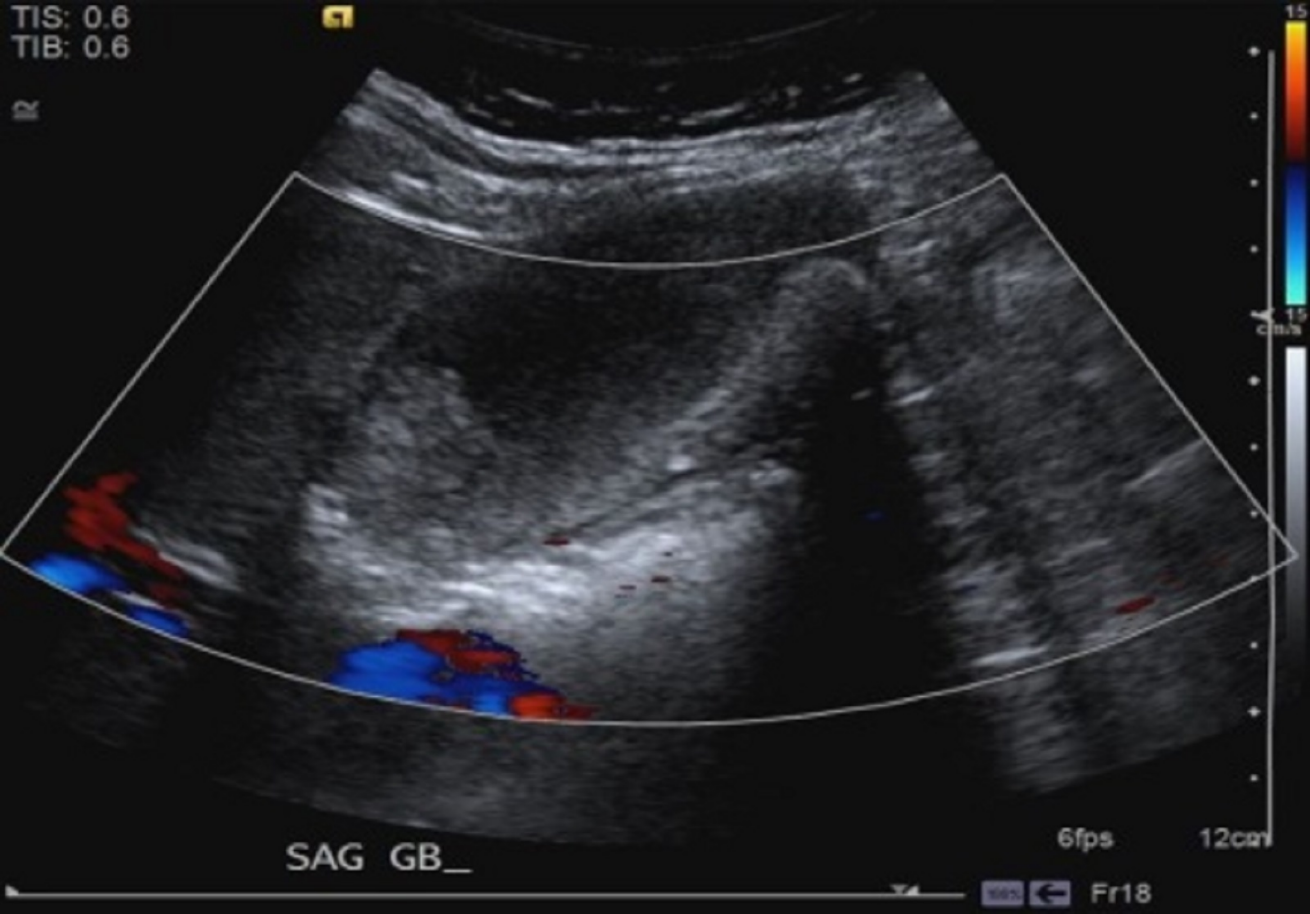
Initial right upper quadrant ultrasound. The image demonstrates no discrete internal flow within the gallbladder with blood flow in the adjacent vasculature.
